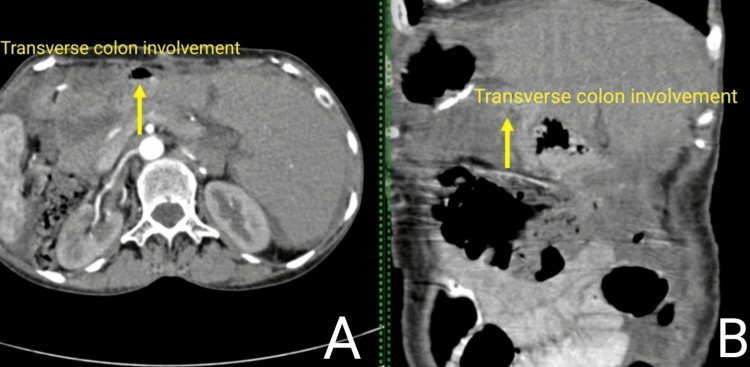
Contrast-enhanced computed tomography (CECT) scan showing involvement of the transverse colon. CECT scan of the abdomen and pelvis showing (A) axial contrast section with the yellow arrow suggesting transverse colon involvement with adjacent bowel wall thickening, and (B) coronal section with the yellow arrow showing transverse colon involvement.
